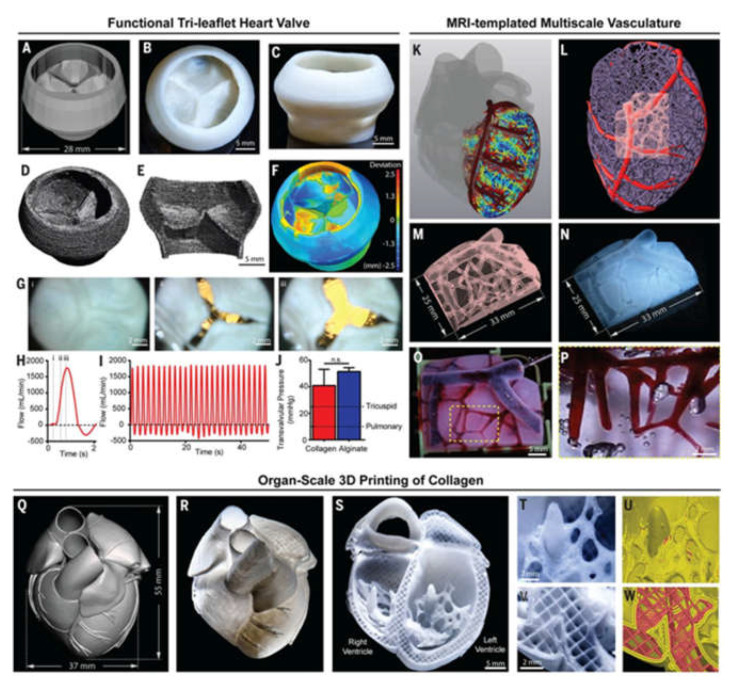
Organ-scale FRESH 3D bioprinting of tri-leaflet heart valve, multiscale vasculature, and neonatal-scale human heart: (A) Tri-leaflet heart valve 3D model at adult human scale; (B,C) Top and side views of FRESH-printed collagen heart valve; (D) Using μCT reconstruction showed the complete printed valve; (E) Lateral cross section of the wall and leaflets; (F) Compared with the 3D model, quantitative gauging of the μCT 3D surface shows average overprinting of +0.55 mm and underprinting of −0.80 mm; (G) Sequence of valve opening under pulsatile flow over 1 s; (H) Doppler flow velocimetry of a single cycle: (i) closed, (ii) half-open, (iii) open; (I) Repeat the cycle in (H); (J) Maximum transvalvular pressure of printed alginate and collagen valves compared to operating pressure for native valves [*N* = 3, data are means ± SD, n. s. indicates *p* > 0.05 (Student t test)]; (K) MRI-derived 3D human heart model (gray) with computationally derived multiscale vascular network shown for the left ventricle. The left anterior descending coronary artery (red) is the template to guide the formation of smaller-scale vessels, which decrease in diameter according to distance from the coronary artery (red to blue); (L) Left ventricle with the left anterior descending artery (red), computationally generated vasculature (purple), and subregion of interest (pink); (M) Transparent subregion showing 3D structure of the vascular network; (N) FRESH-bioprinted with collagen, showing reproduction of the vascular network; (O) The vascular network was perfused with red glycerin; (P) The collagen was optically removed and perfused with red glycerol to a diameter of 100-μm blood vessels; (Q) MRI-derived 3D human heart scaled to neonatal size; (R) FRESH-printed collagen heart; (S) A printed cross-sectional view of the collagen heart showing the left and right ventricles and the internal structure; (T,U) High-fidelity image of the trabeculae in the left ventricle (T) showing reproduction of the complex anatomical structure from the G-code (U); (V) A high-definition image of the septal wall between ventricles (W) Showing reproduction of the square-lattice infill [173].
